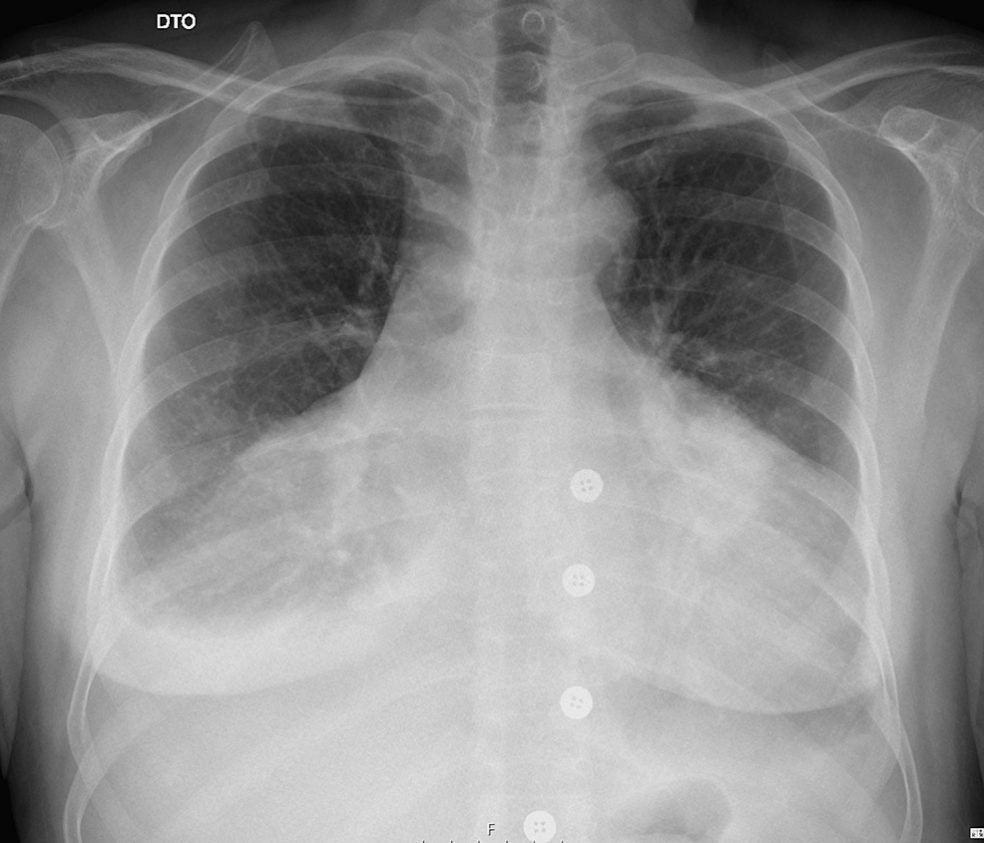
CXR highlighting the patient’s exuberant cardiomegaly. CXR: chest X-ray
